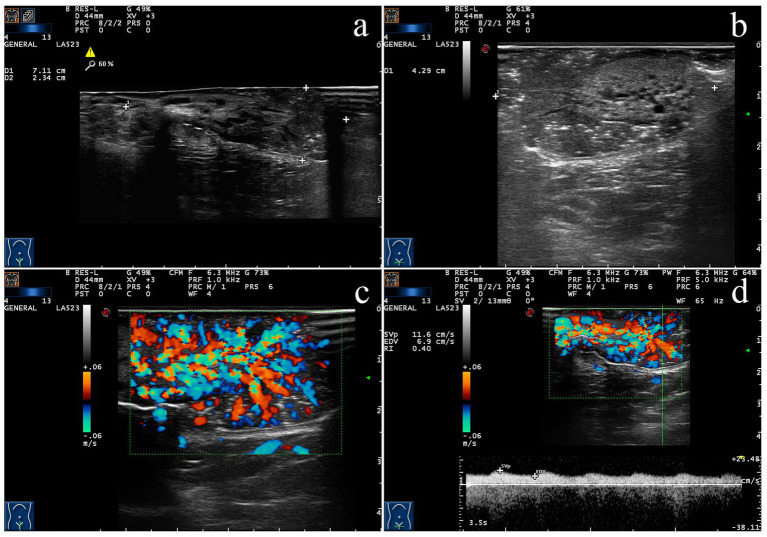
Ultrasound findings of giant vulva VX in a child. (a) The maximum cross-sectional image of the lesion under two-dimensional (2D) ultrasound. (b) Image of the perpendicular cross-section to the maximum cross-section of the lesion under 2D ultrasound. Multiple punctate hyperechoic foci and several strip-like anechoic areas were observed within the hypoechoic lesion. (c) CDFI demonstrated abundant blood flow signals inside the mass, presenting a “fiery sea sign-like” pattern. (d) Pulsed Doppler ultrasound reveals low-velocity and low-resistance blood flow within the lesion.
